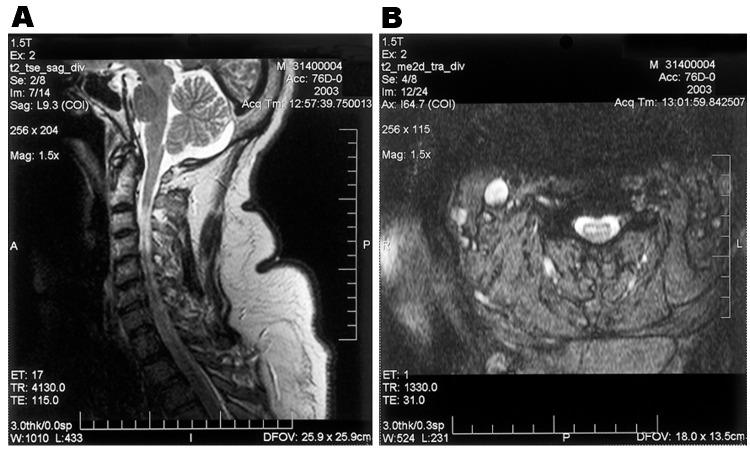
Saggital (A) and axial (B) T2-weighted magnetic resonance images of the cervical spinal cord in a patient with acute asymmetric upper extremity weakness and subjective dyspnea. A shows a diffuse cervical cord signal abnormality, and B shows an abnormal signal in the anterior horn region.
